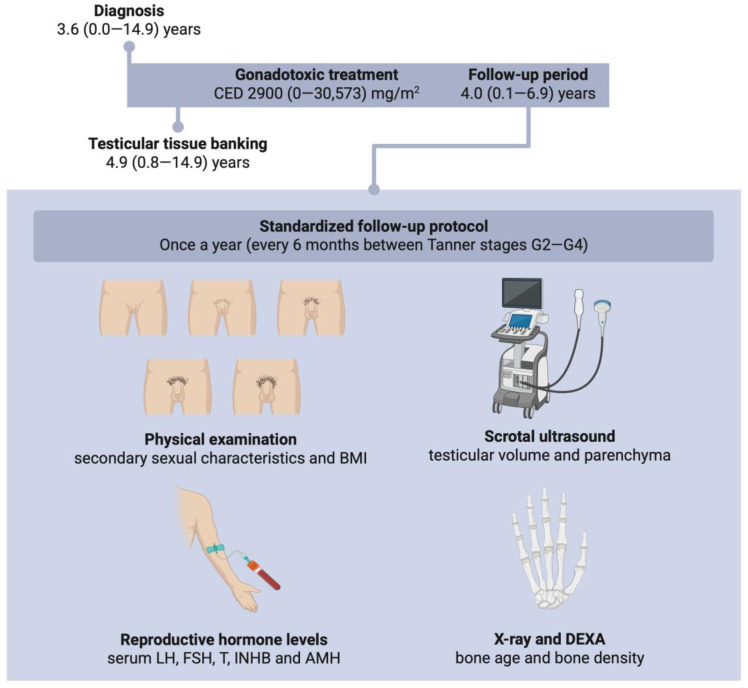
Overview of the study design and follow-up assessments to evaluate pubertal development after paediatric gonadotoxic treatment. This figure outlines the study design and follow-up assessments, which include physical examinations by a fertility specialist, scrotal ultrasounds, reproductive hormone measurements, X-rays, and dual-energy X-ray absorptiometry (DEXA) scans. Created in BioRender. Goossens, E. (2026) https://BioRender.com/4dxrx6v (accessed on 20 February 2026).
